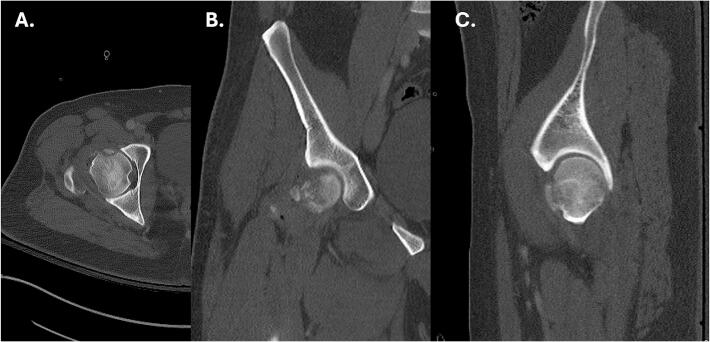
CT of the right hip demonstrating axial (A), coronal (B), and sagittal (C) views revealing a right femoral head fracture.
